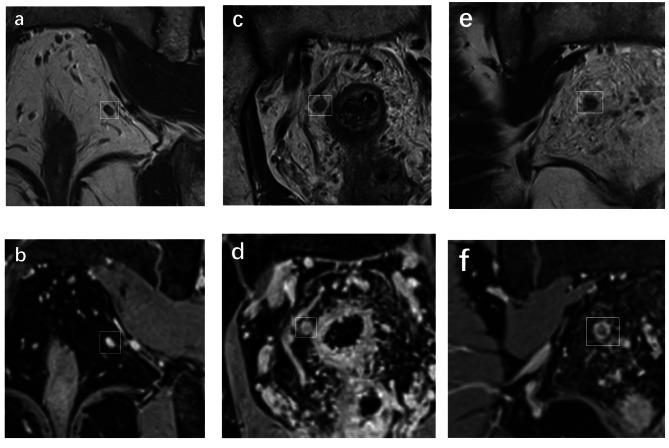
Coronal non-fat-suppressed high-spatial-resolution T2-weighted imaging (a, c and e); coronal fat-suppressed isotropic contrast-enhanced three-dimensional high-spatial-resolution T1-weighted imaging (b, d and f). The lymph nodes were signed by white boxes. a-b, Benign node with 3.7 mm in short-axis diameter, showed oval, smooth border, homogeneous signal and homogeneous enhancement. c-d, Benign node with 5.6 mm in short-axis diameter, showed round, irregular border, heterogeneous signal and heterogeneous enhancement. e-f, Malignant node with 5.8 mm in short-axis diameter, showed round, irregular border, heterogeneous signal and heterogeneous enhancement
